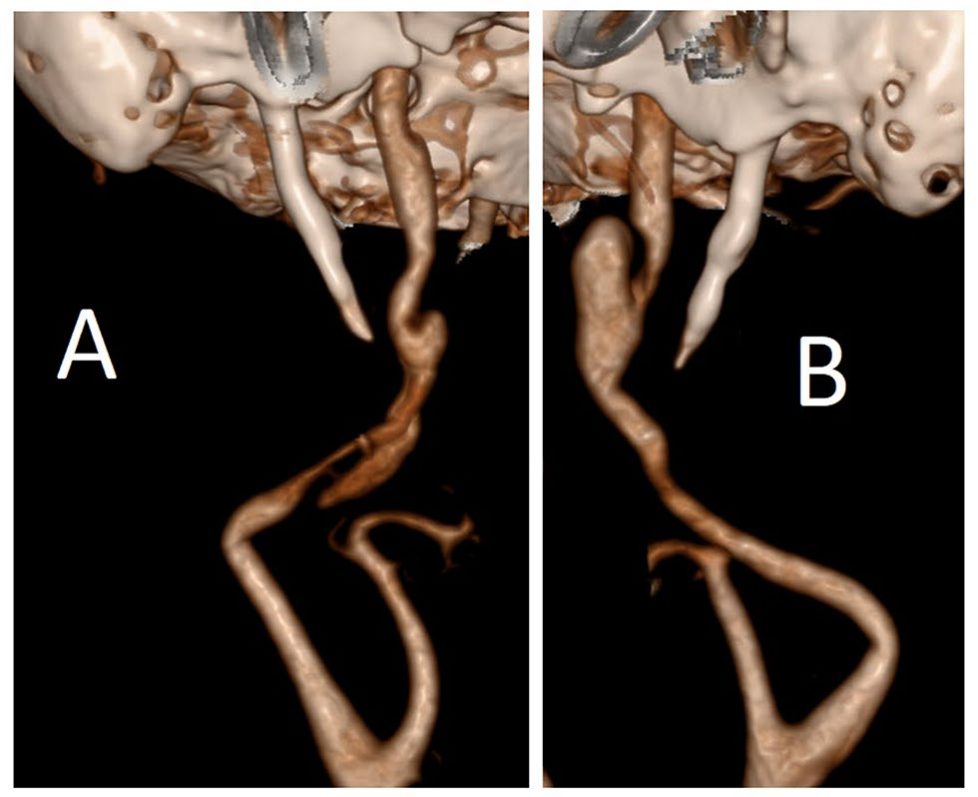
3D reconstruction of the CT-angiography demonstrating the ICA-D of the right (A) and left (B) side with short SP-ICA distance. CT– computed tomography, ICA-D– internal carotid artery dissection, ICA– internal carotid artery, SP– styloid process
